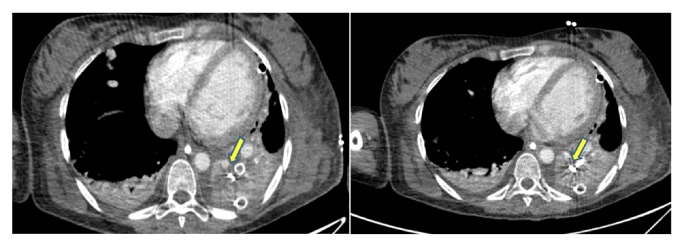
Chest CT showing signs of pAVM embolization and pulmonary resection of the LLL.
